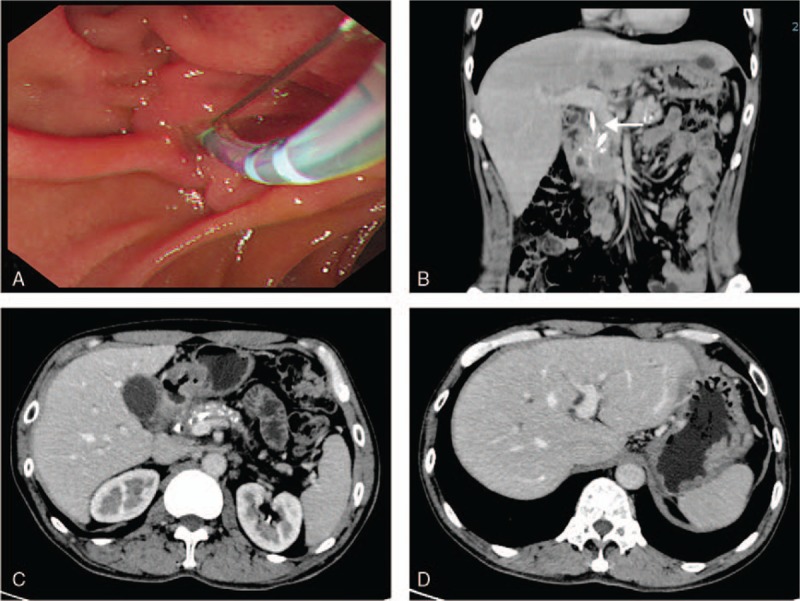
Intraoperative photo of endoscopic transpapillary drainage and abdominal CT taken after operation. A, ERCP shows opening of major duodenal papilla was normal. B, Coronal CT scan reveals the bile duct stent and the pancreatic duct stent. C, CT scan shows no cystic lesion in the head. D, CT scan reveals cystic lesion next to tail of the pancreas was disappeared 6 weeks after operation. CT = computed tomography, ERCP = endoscopic retrograde cholangiopancreatography.
